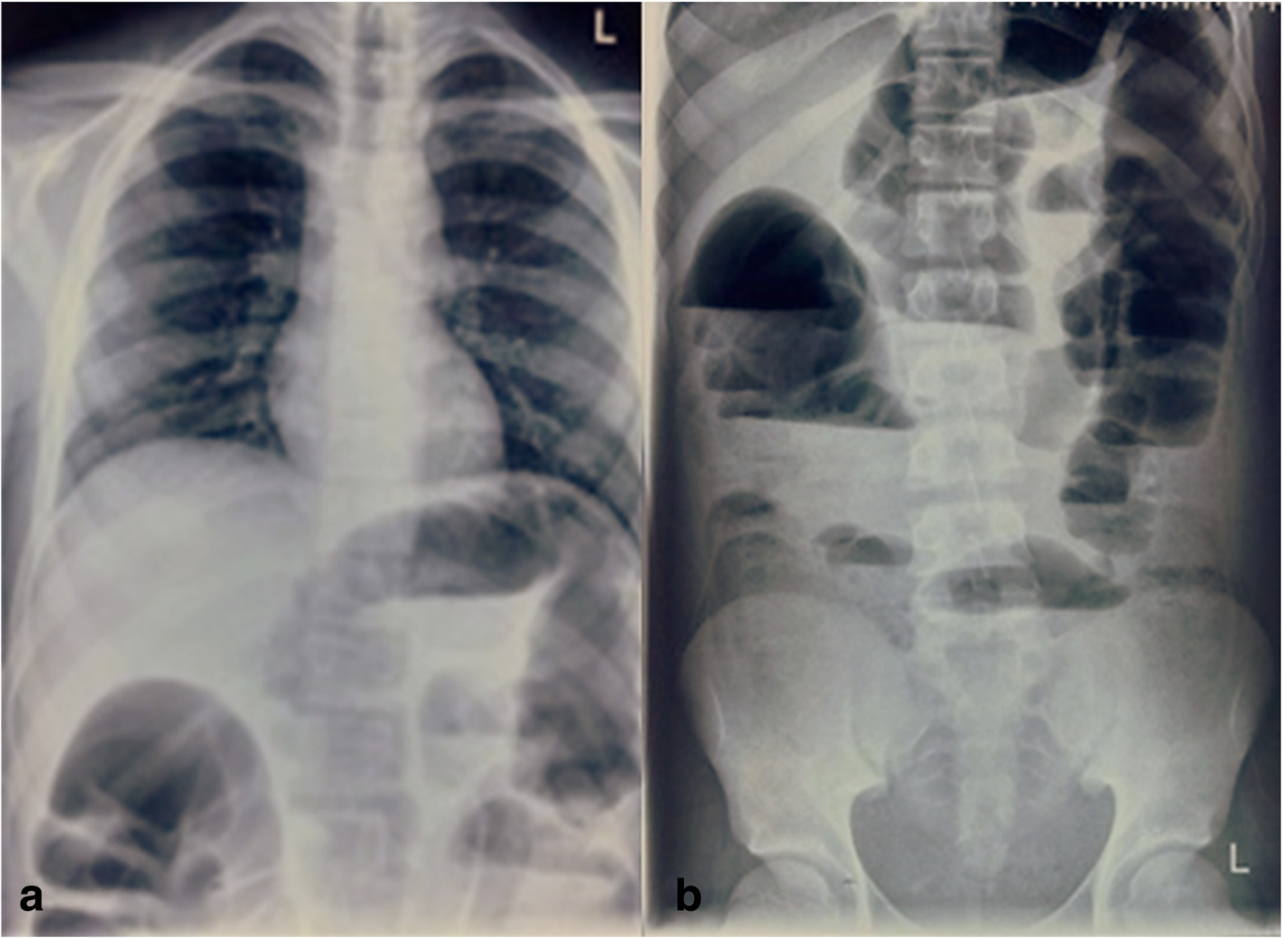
a Chest X-ray posteroanterior view; b abdominal X-ray anteroposterior erect view
